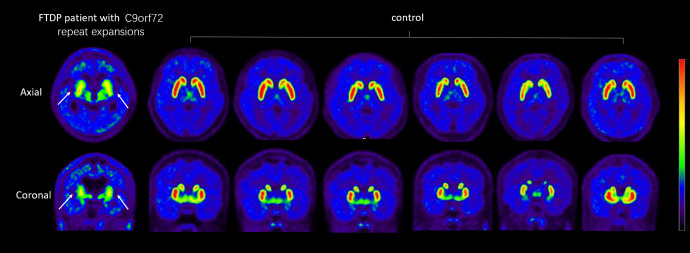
Reduced dopamine terminal in FTPD patients with C9orf72 expansion. DTBZ-PET/CT imaging of the VMAT2 distribution in the FTDP patient (III-6) with C9orf72 expansion and controls (*n* = 6). The same slice is presented for all participants. White arrows indicate obvious dopamine decline of the bilateral striatum in the patient. Note the symmetric decline of vesicular transporter availability without a posterior-to anterior gradient.
